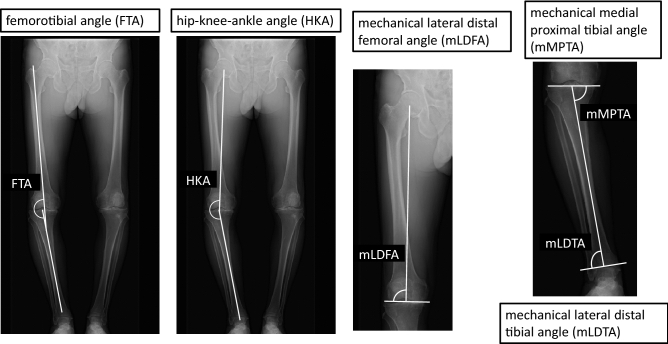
Radiographic measurements of coronal lower extremity parameters. Image shows the femorotibial angle (FTA) which is the lateral angle at the intersection between the femoral bone axis and the tibial bone, the hip–knee–ankle angle (HKA) which is the lateral angle between the mechanical axis of the femur and the tibia), the mechanical lateral distal femoral angle (mLDFA) which is the lateral angle between the mechanical axis of the femur and the distal femur joint line, the mechanical medial proximal tibia angle (mMPTA) which is the medial angle between the mechanical axis of the tibia and proximal tibia joint line, and the mechanical lateral distal tibia angle (mLDTA) which is the angle between the tibial mechanical axis and distal tibial joint surface.
